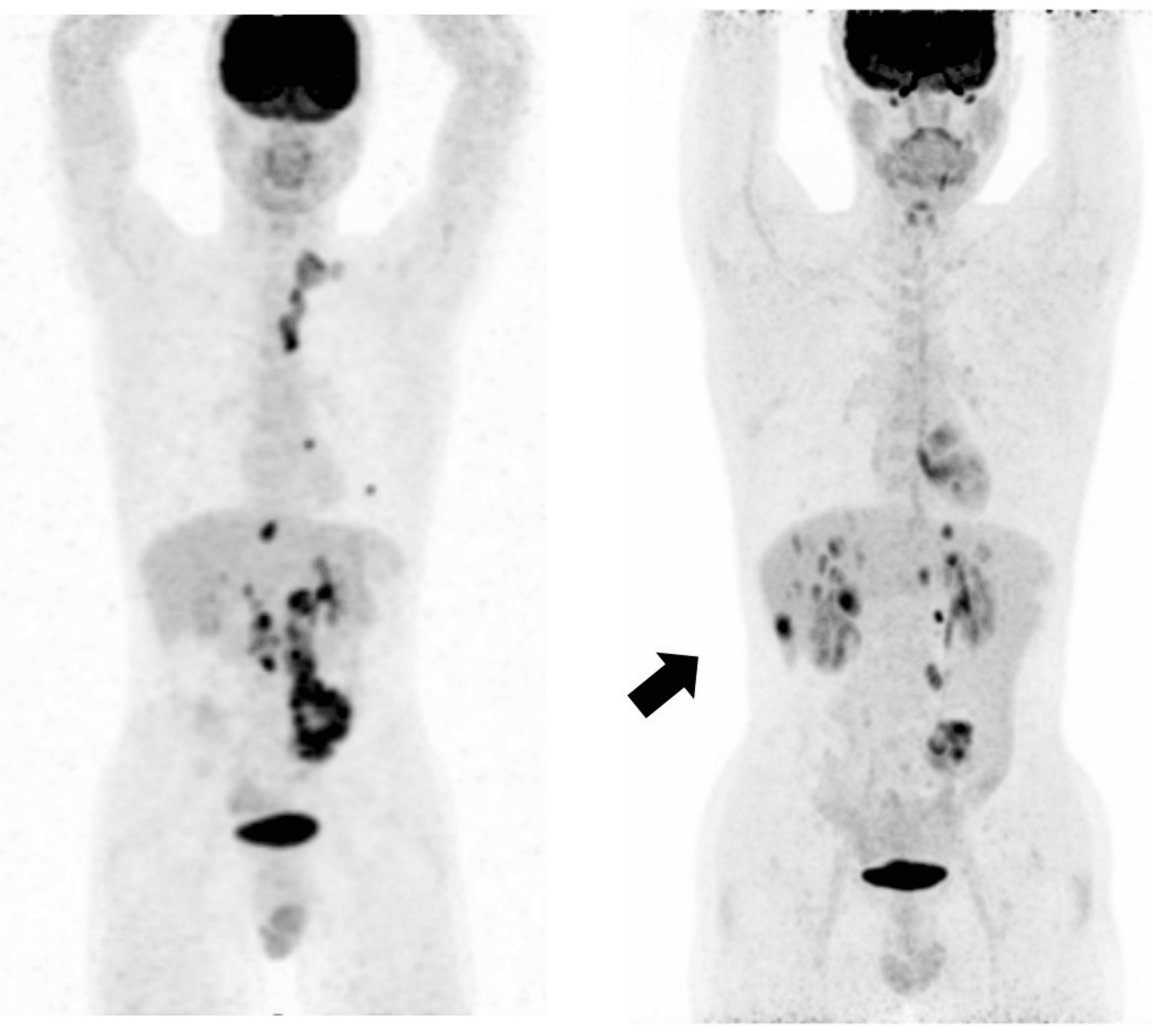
PET/CT findings of the patient. Residual abnormal accumulation was observed in the SD junction with SUV max 13.08, para-aortic lymph node 11.53, and mesenteric lymph node 7.54. Multiple liver metastases were observed, including SUV max 10.87 in S4, 9.39 in S2, and 7.67 in S8. SUV: standardized uptake value
